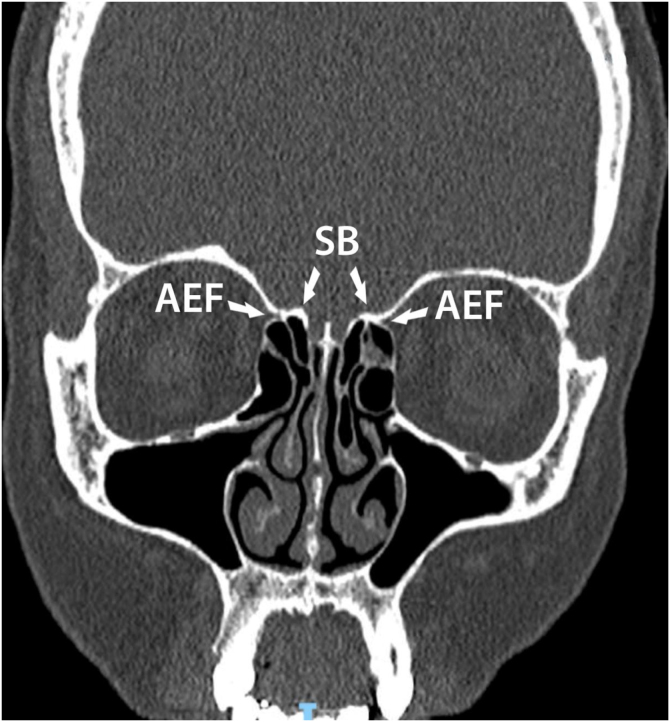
Computed tomography in coronal reconstruction showing anterior ethmoidal artery adhered to the skull base. SB, Skull Base; AEF, Anterior Ethmoidal Foramen.
